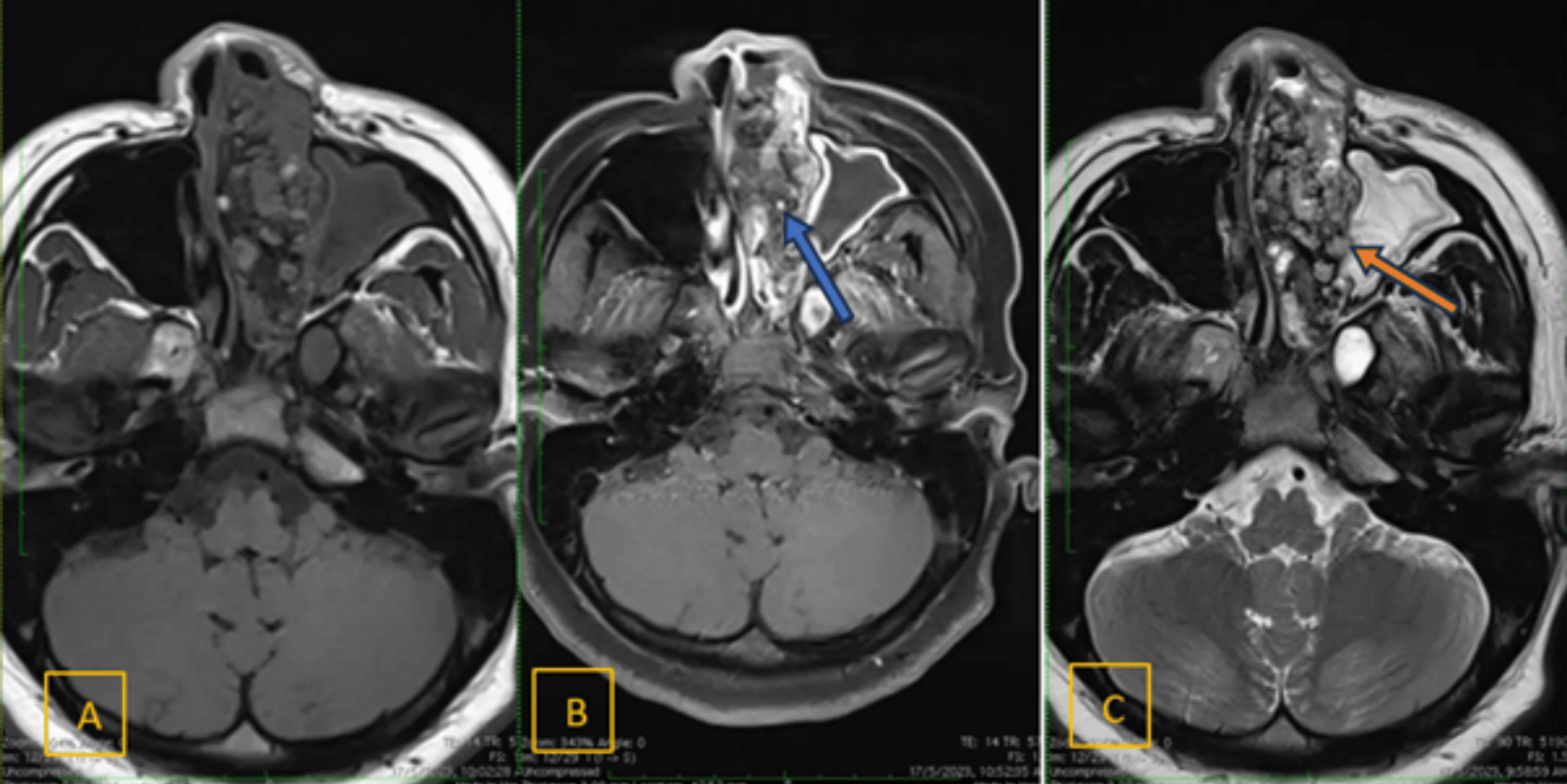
MRI axial view (A) T1-weighted pre-contrast. (B) T1-weighted post-contrast. (C) T2-weighted. Blue arrow: heterogeneous enhancement mass occluding the left nasal passage. Multiple intratumoral calcifications and T1 hyperintense signals due to proteinaceous fluid or hemorrhagic components. Orange arrow: erosion of the left medial wall of the maxillary sinus MRI: magnetic resonance imaging
